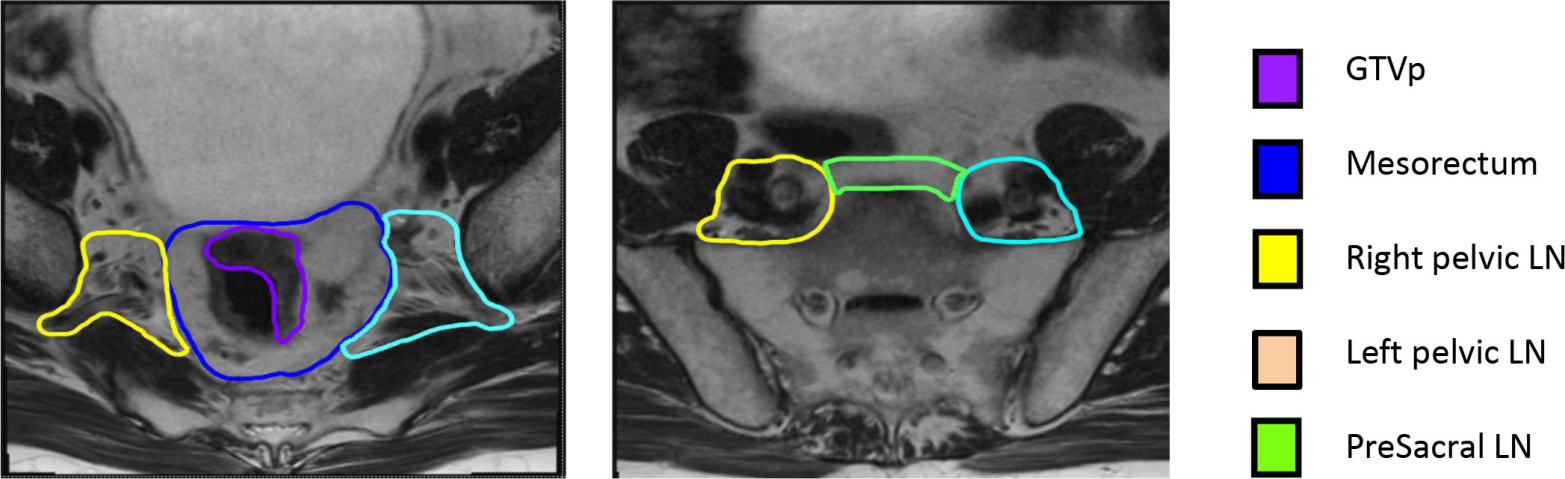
Example contoured structures on 2D-MRI. GTVp, gross tumour volume; LN, lymph node.
